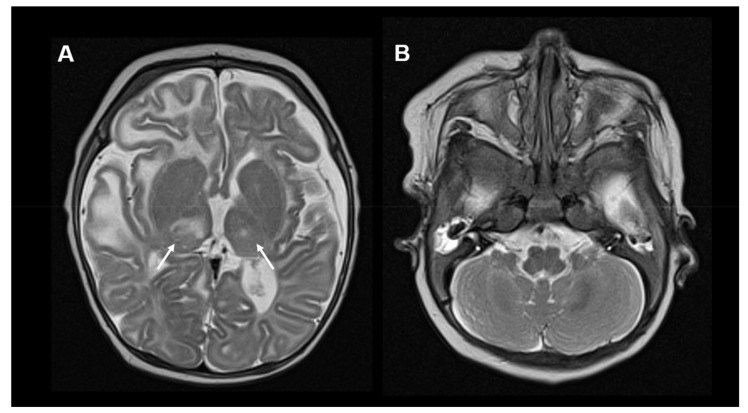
Neuroimaging in HPDL deficiency: (A) Brain MRI, T2-weighted images of a 3-month-old girl with HPDL deficiency. Images show subcortical T2-hyperintensities, mainly affecting the right frontotemporal regions. Moreover, asymmetrical signal abnormalities of the thalami are visible (white arrows). (B) No cerebellar abnormalities are visible.
